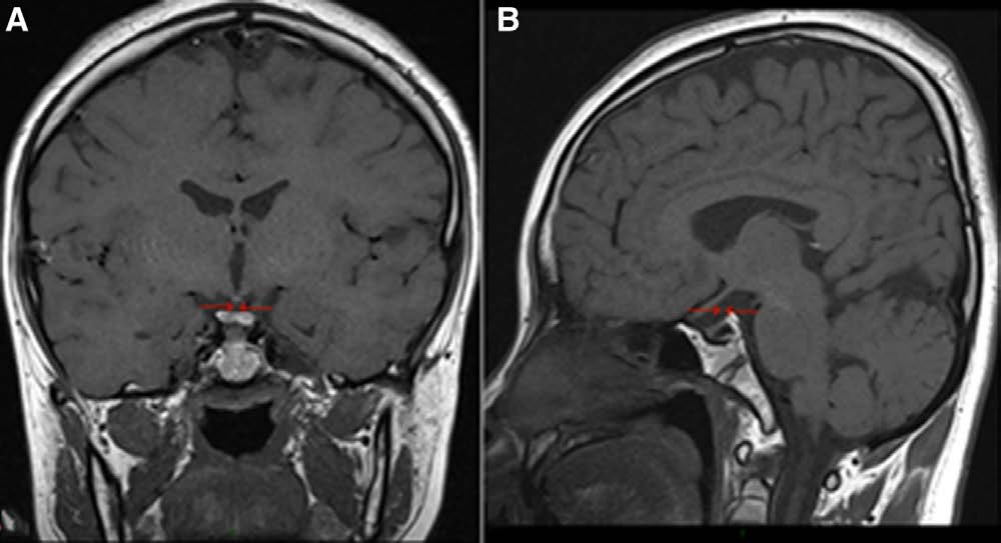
Pituitary stalk thinning: MRI scan of the pituitary gland (A: coronal, B: sagittal): the pituitary gland measures approximately 4 mm in height, with a thinned and poorly defined pituitary stalk. The high-signal neuropil is located superiorly to the dorsal aspect of the saddle. MRI = magnetic resonance imaging.
